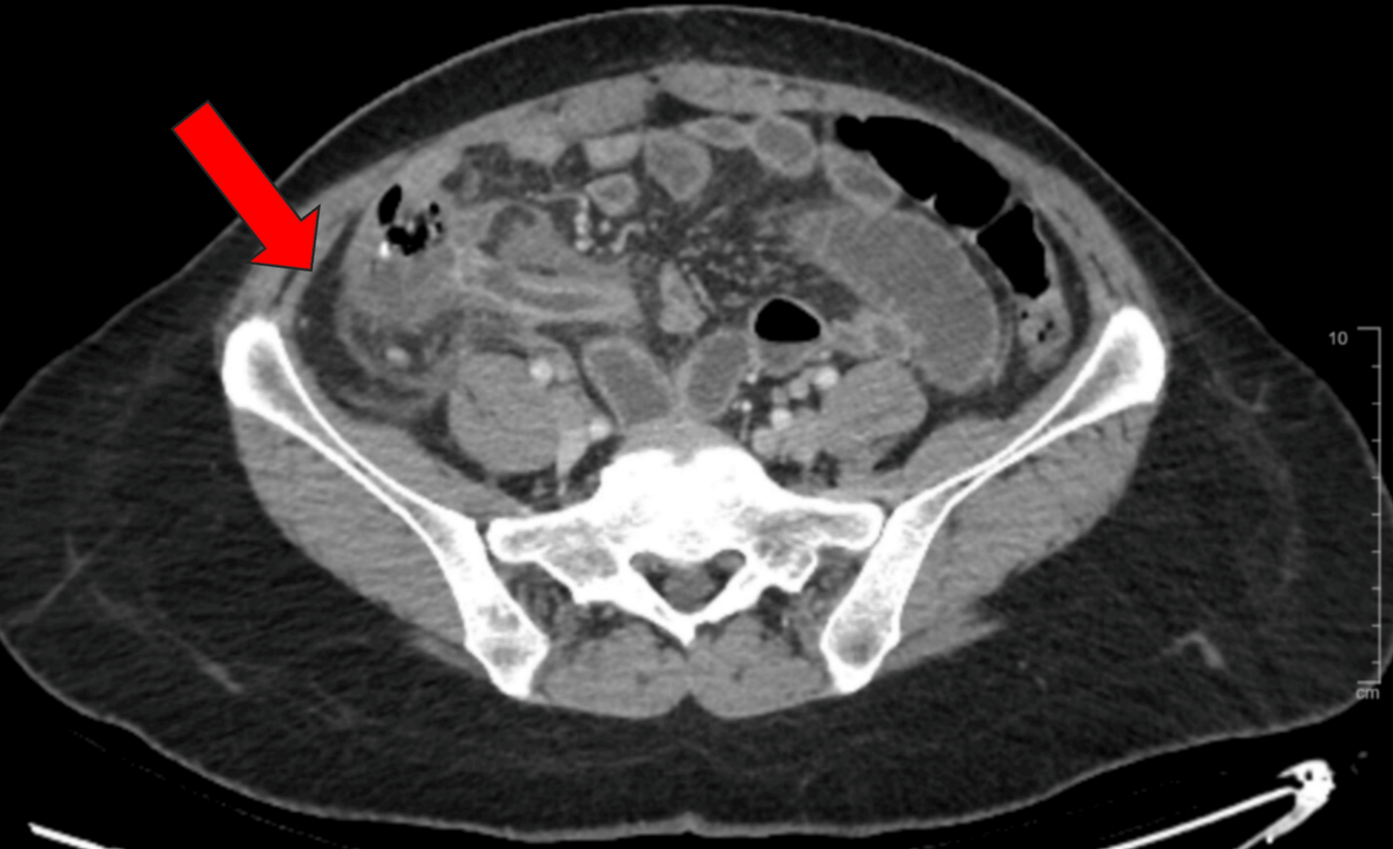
Computerized tomography (CT) imaging of the abdomen and pelvis with IV contrast demonstrating a dilated appendix measuring 1.2 cm with diffuse mucosal thickening and surrounding inflammatory changes. Arrow size: 2.5 cm x 0.6 cm
